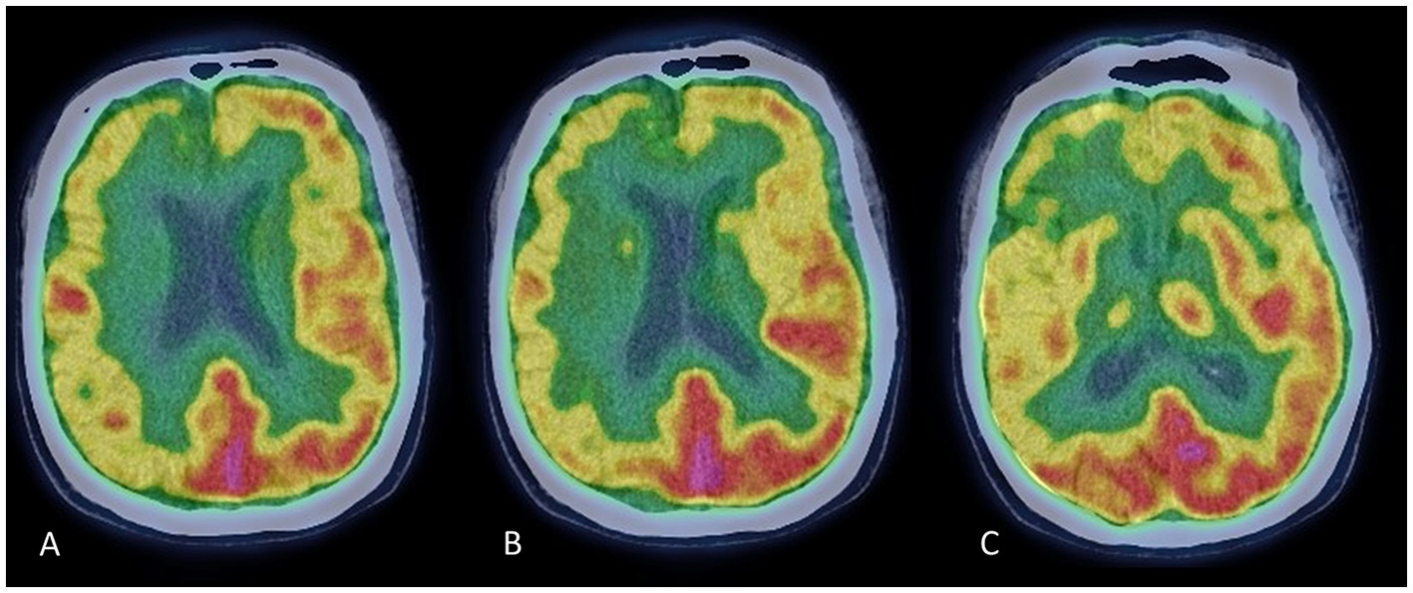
Brain FDG-PET scans at three rostrocaudal levels (A–C). Images show a pattern of diffuse hypometabolism involving the right frontal and frontotemporal cortex, which is more evident in the medial regions.
